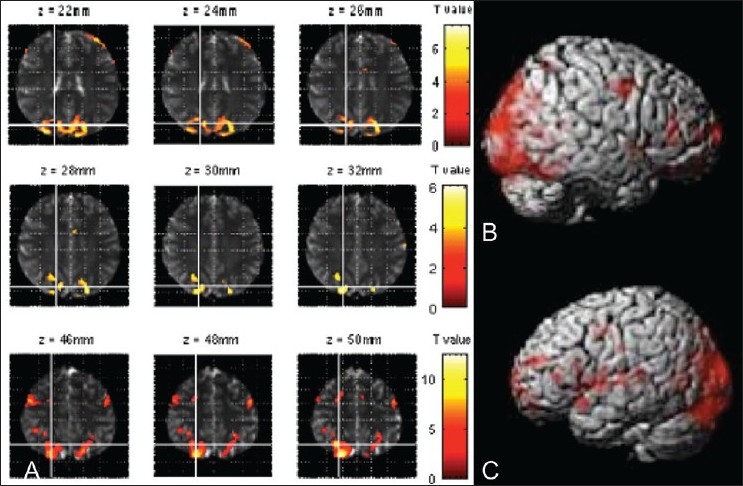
MRI images (A) show the cortical activation pattern for the whole sample for postlexical access semantic association task. Functional scans were done in a 1.5-T whole-body scanner and overlaid on an SPM anatomical template. Surface rendering of cortical activation for word concept association for all subjects for the right (B) and left (C) hemispheres (FWE-corrected, *P*≤0.001).
